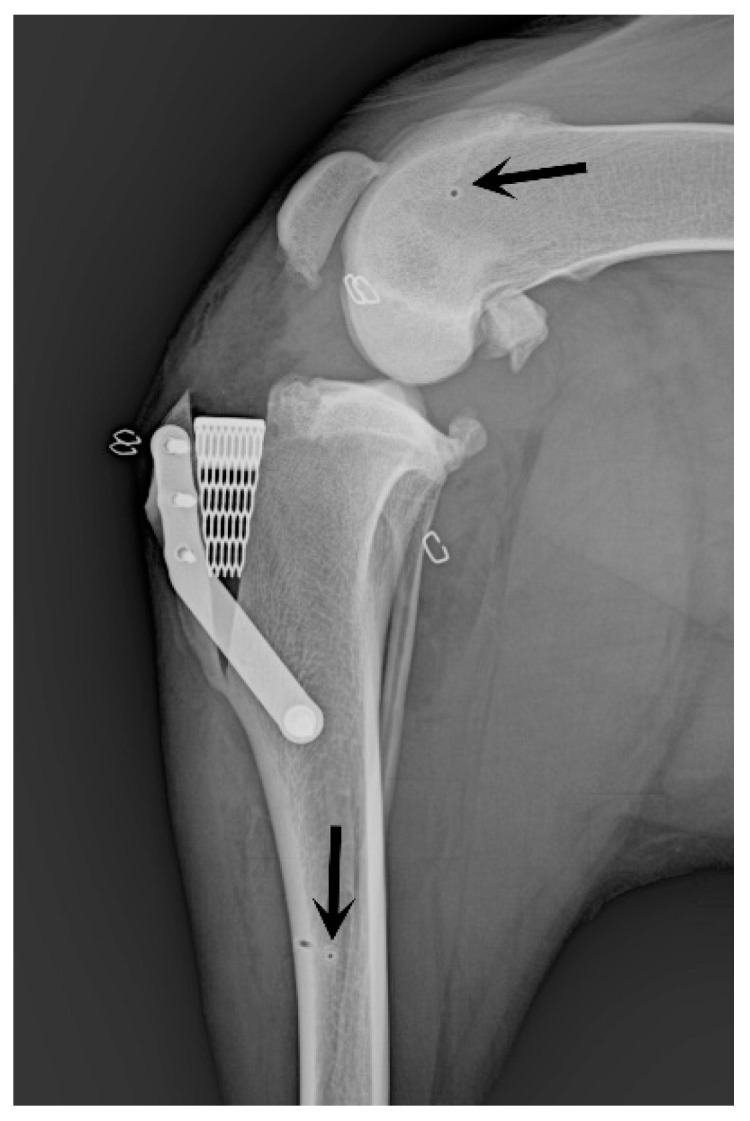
Postoperative X-Porous TTA mediolateral radiographic projection. Note the small holes due to the K wires of the traction stirrups connected to the Titan distractor.
